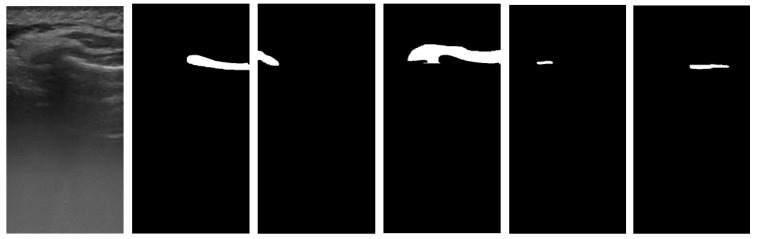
From left to right: original ultrasound image, bone, crown, gingiva, pocket, root.
